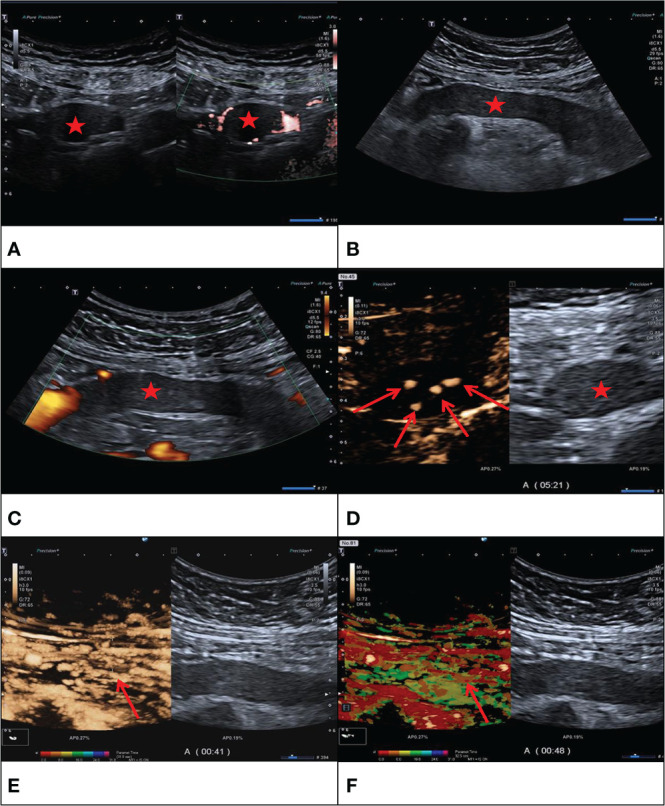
F/43Y, solid cast lesion, (A, B) no “sieve hole sign” and “multi-track sign” in conventional ultrasound (Red star), (C) no blood flow signal detected in the lesion (Red star), (D, E) CEUS showed the CEUS manifestations of “sieve hole sign” and “multi-track sign” respectively (Red arrow). (F) Contrast-enhanced ultrasound parametric imaging shows the presence of multi-track sign (Red arrow). The pathological diagnosis is IVL in this case.
